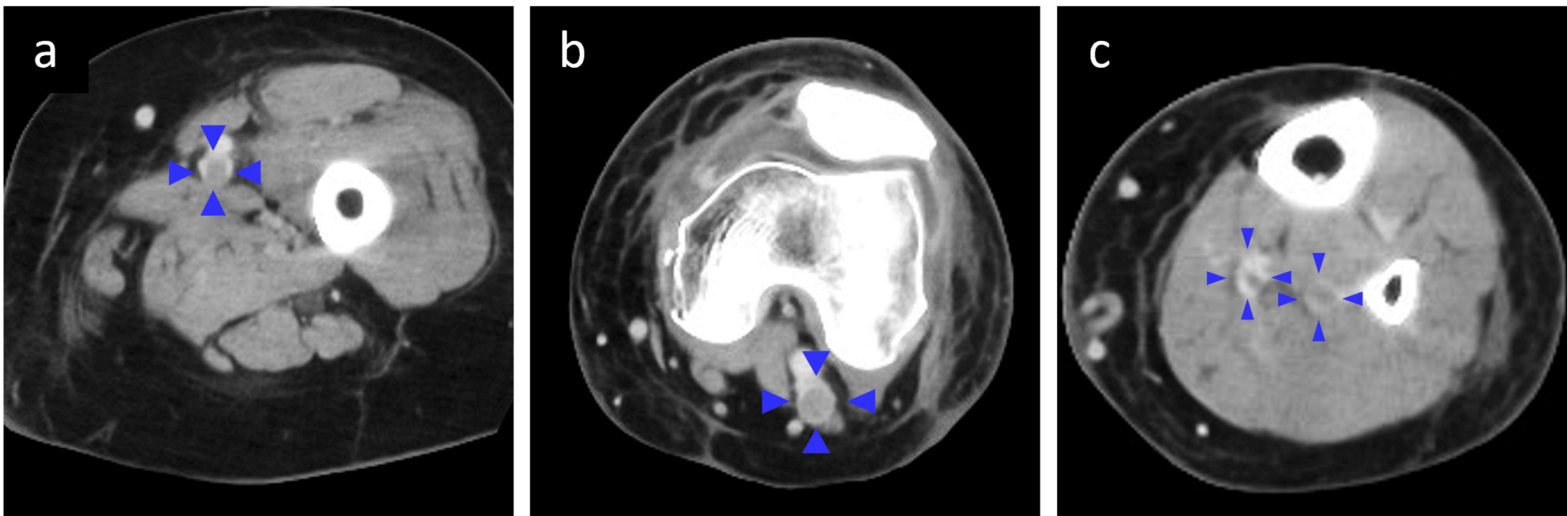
Contrast-enhanced CT axial views of the left leg with VTE at seven days post-operation (a) Femoral vein (enclosed by blue arrowheads). (b) Popliteal vein (enclosed by blue arrowheads). (c) Soleal veins (enclosed by blue arrowheads). VTE, venous thromboembolism
